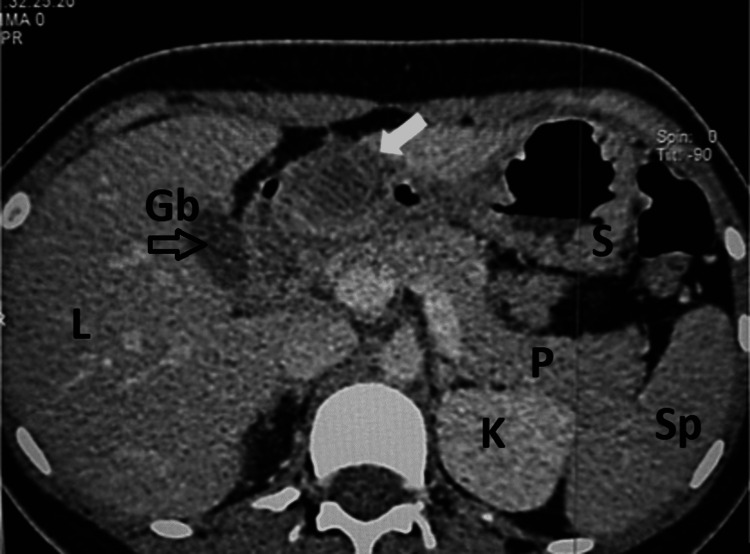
Axial computed tomography (CT) scan of the abdomen. The image shows a cystic mass in the antrum (white arrow) corresponding to the lesion seen on endoscopy. Liver (L), gallbladder (Gb indicated by open black arrow); body of stomach (S), spleen (Sp), pancreas (P), and left kidney (K).
